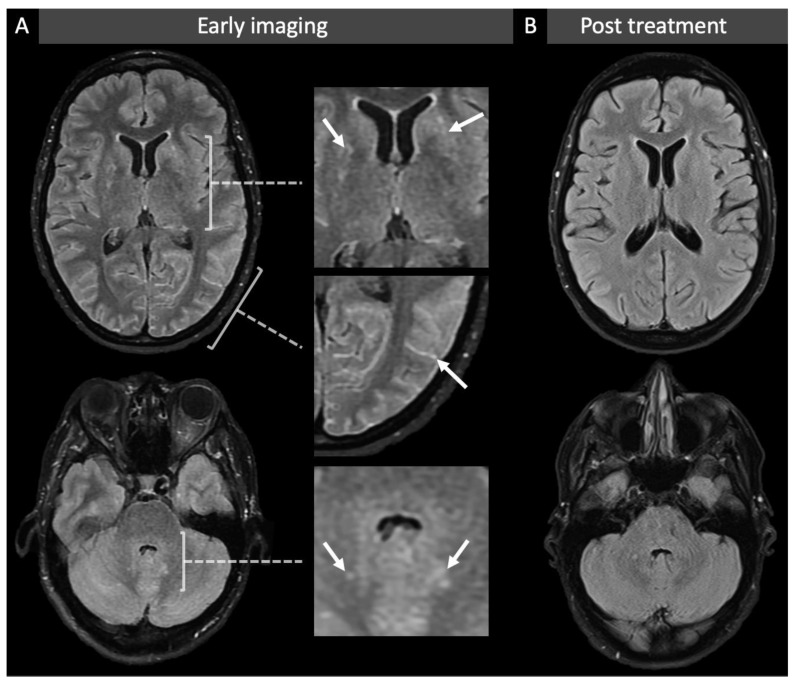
MR images before and after treatment. Panel (A): Axial MRI FLAIR images of the supratentorial (top row) and infratentorial (bottom row) brain at nadir (day 12 from symptom onset) demonstrate abnormal, ill-defined, high T2w signal in both basal ganglia (top magnified image) and dentate nuclei (bottom magnified image) in a perivascular distribution. Abnormal intra-sulcal FLAIR hyperintensity is also noted at this time (middle magnified image). Panel (B): Repeat axial FLAIR images following treatment (day 33 from symptom onset) demonstrate resolution of the abnormal signal in the deep ganglionic structures and sulcal spaces.
